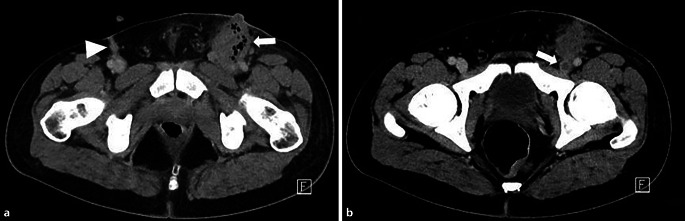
Kontrastmittelgestützte Computertomographie (CT) des Beckens im Weichteilfenster. a Links inguinal lässt sich ein Abszess (*Pfeil*) ventral der Gefäße mit Ausbreitung bis zur Kutis abgrenzen. Auf der Gegenseite finden sich rechts inguinal ebenfalls ventral der Gefäße narbig imponierende Veränderungen (*Pfeilspitze*). b Zusätzlich ist in der linken V. femoralis communis eine Thrombose abzugrenzen (*Pfeil*)
